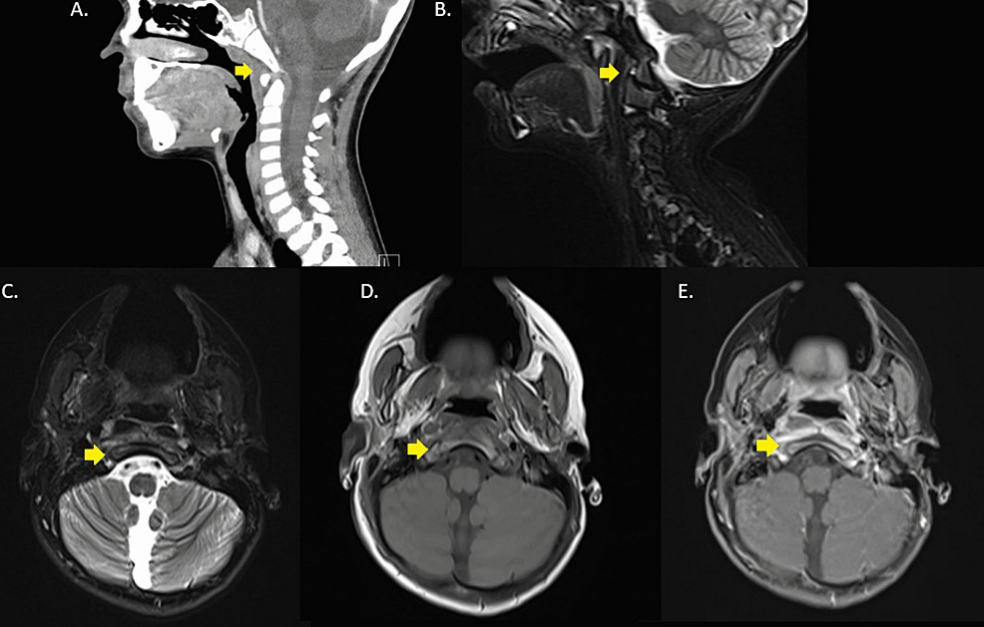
Grisel syndrome (A) Sagittal section contrast-enhanced CT of the neck; (B) Sagittal section T2-weighted image with fat suppression of the cervical spine; (C) Axial section T2-weighted image with fat suppression of the skull base; (D) Axial section T1-weighted image pre-contrast of the skull base; (D) Axial section T1-weighted image post-contrast of the skull base.
